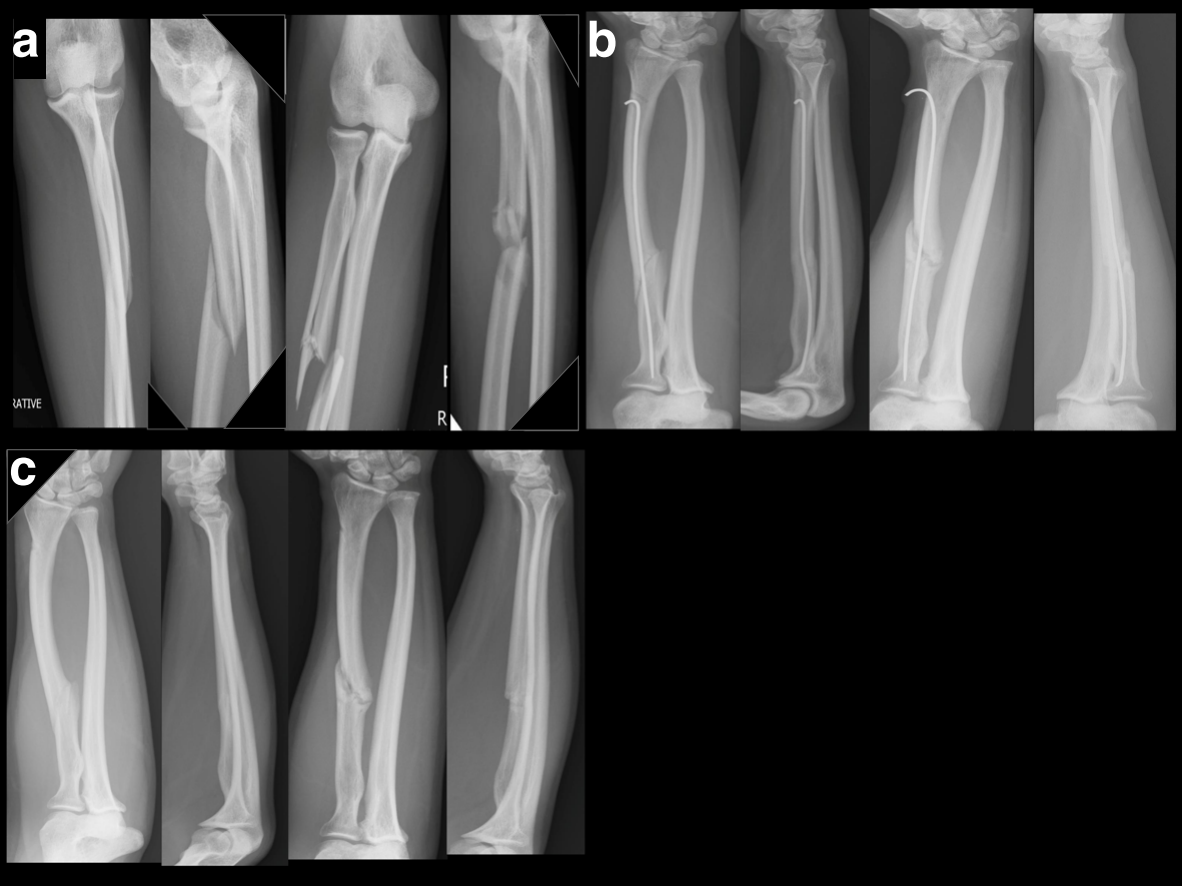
A 30-year-old male was involved in a traffic accident and sustained bilateral proximal radial shaft fractures. a Preoperative anteroposterior radiographs show bilateral proximal radial oblique diaphyseal fractures. b Six months postoperative views show TENs placed to stabilize the radial shaft fractures. Bridging callus is noted around the fractures sites. c Postoperative follow-up radiographs reveal bone union of bilateral proximal radial shaft fractures. Removal of the implants 8 months postoperation did not contribute to refractures
